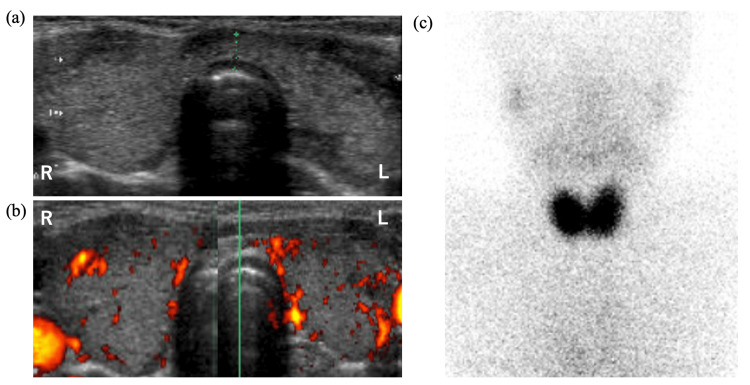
Ultrasound images of B-mode (a) and color Doppler (b) at six years of age when Hashimoto thyroiditis (HT) was diagnosed and a thyroid scan with 99mTc (c) at seven years of age when Graves disease (GD) was diagnosed in case 1.
